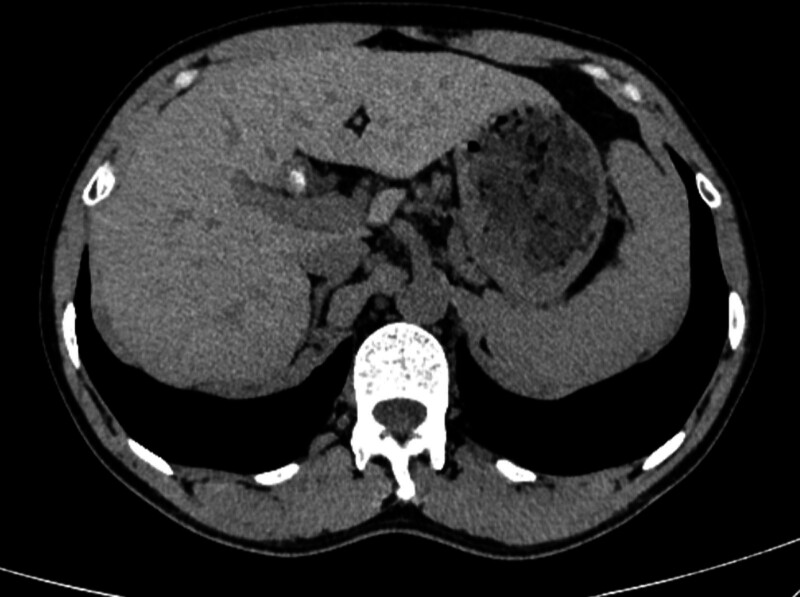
CT showed high density shadow in the repeated gallbladder. CT = computed tomography.
